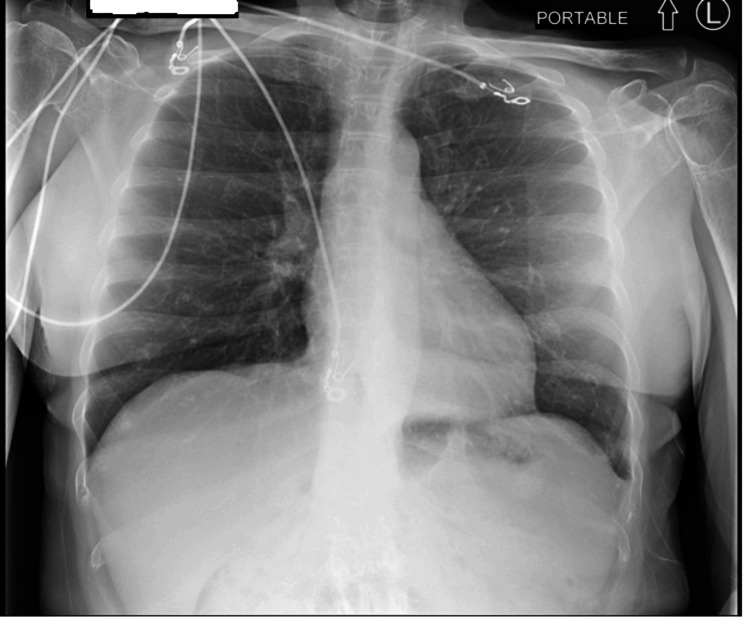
Antero-posterior chest X-ray image obtained on the day of presentation to the Emergency Department, showing no acute cardiopulmonary abnormalities.
